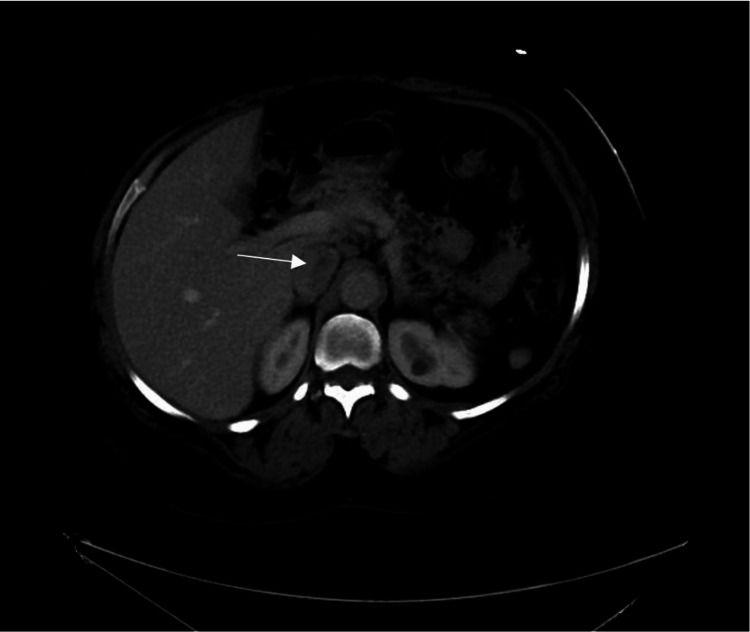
Abdominal CT scan with IV contrast demonstrating possible thrombus (marked by arrow)
